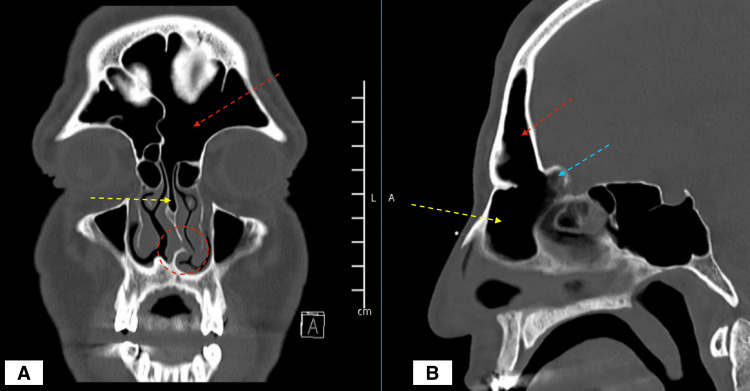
CT scan of the paranasal sinuses; A: coronal cut; B: sagittal cut red arrow: the left frontal sinus; yellow arrow: pneumatized anterior nasal septum; blue arrow: pneumatised crista galli; red circle: left nasal septal spur
